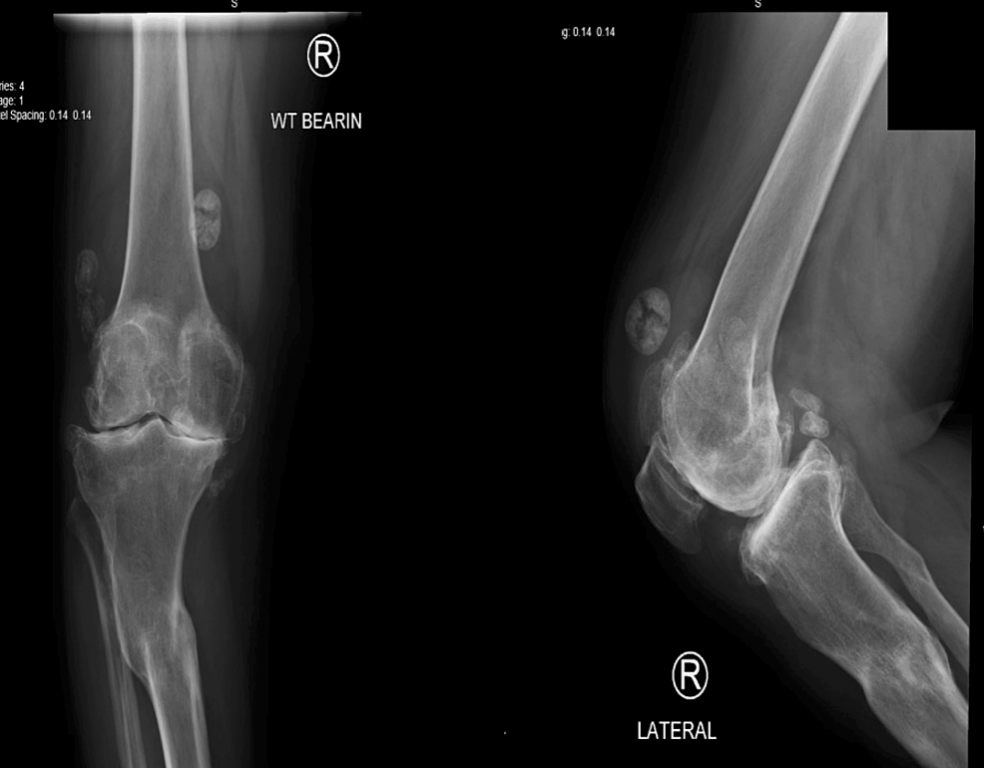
Radiograph showing tibial varus deformity
